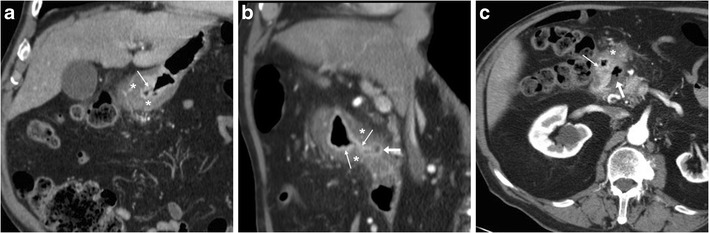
An elderly 87-year-old male underwent emergency contrast-enhanced CT to investigate acute abdominal pain with epigastric tenderness but no peritonism: the antropyloric region showed pronounced, stratified mural thickening with oedematous submucosa (*), mucosal hyperenhancement best seen in arterial-phase images (thin arrows), and a posterior prepyloric deep ulcer crater (arrows) filled by fluid and air, which was subsequently confirmed by endoscopy
